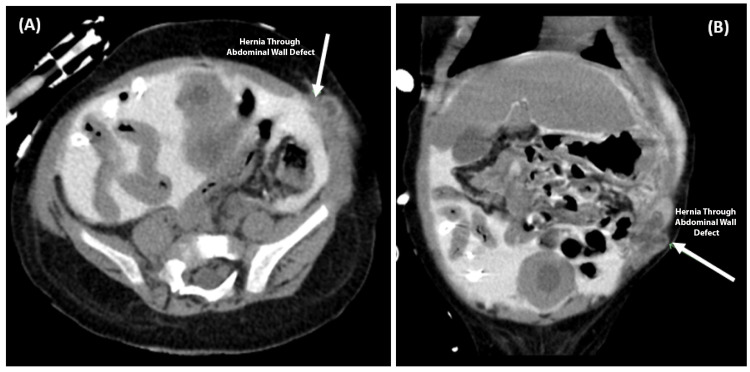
CT scan revealing an abdominal wall hernia at the site of a previous colostomy. Images are in the axial (A) and coronal (B) planes. Contrast was injected through the peritoneal dialysis catheter to determine areas of fluid accumulation that could explain the filling and emptying dysfunction noted during dialysis. The white arrows signify the abdominal wall hernia.
